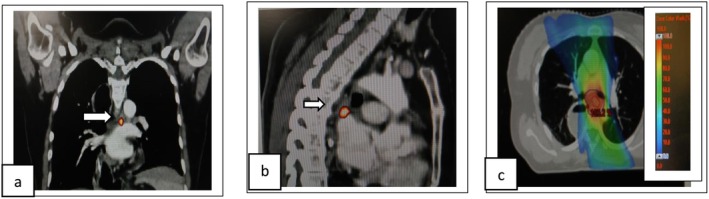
First recurrence in mediastinal nodal region. (a, b) White arrow in coronal and sagittal sections of CT superimposed with PET shows first mediastinal nodal recurrence. (c) Axial CT section shows dose color wash of mediastinal nodal treatment volume.
